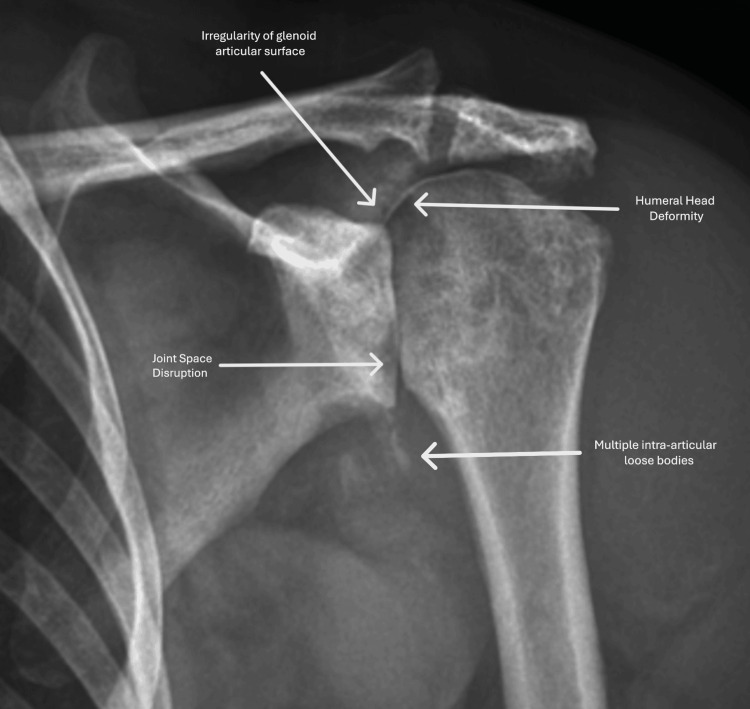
External rotation radiograph of the left shoulder demonstrating advanced destructive changes of the glenohumeral joint, including humeral head deformity, irregularity of the glenoid articular surface, joint space disruption, and multiple intra-articular loose bodies, consistent with Charcot neuropathic arthropathy.
